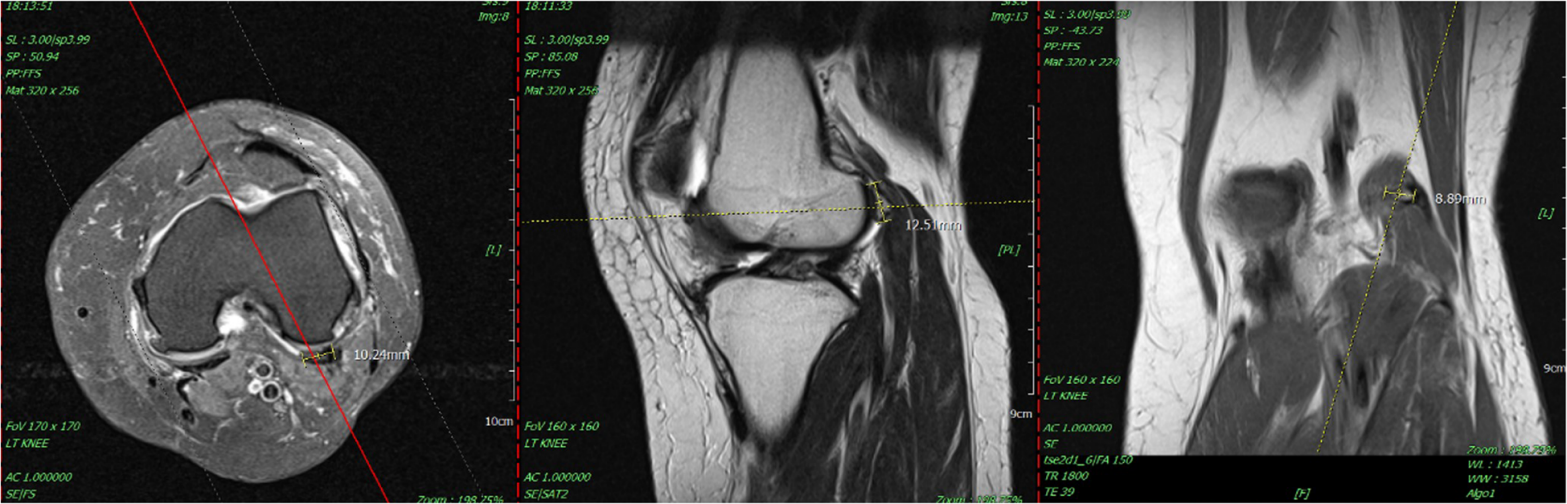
The left knee MRI showed a joint intruded fabella with tear of lateral meniscus in Sagittal view
